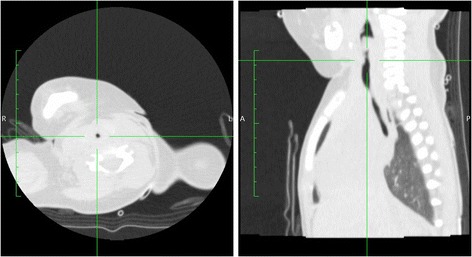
Neck CT images taken at POD 2. Subglottic stenosis of the trachea was detected. Tracheal diameter at the narrowest level was calculated as 3.2 mm. *Left*, transverse image of the neck. *Right*, sagittal image of the neck
